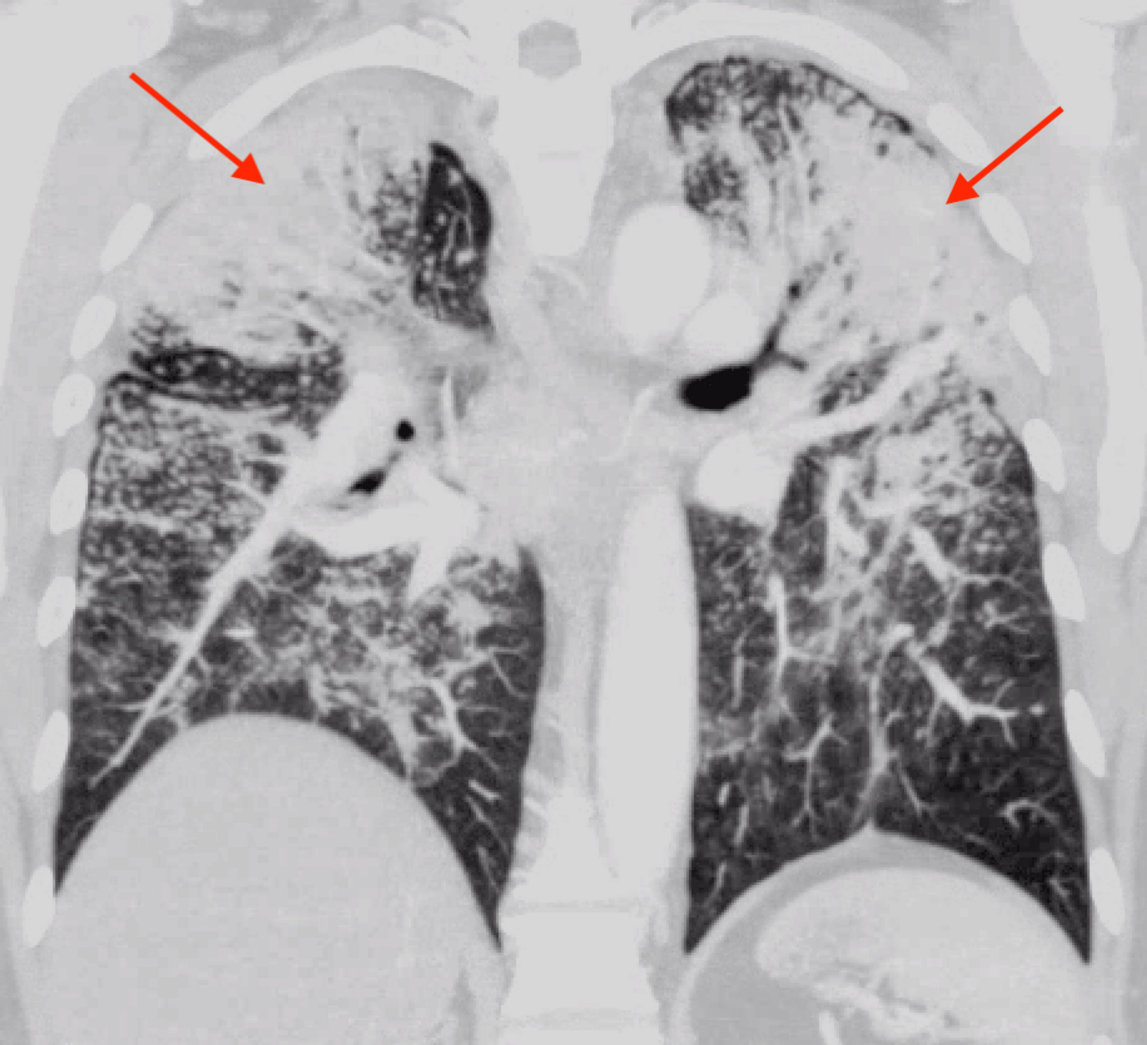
Coronal CT chest view at admission
